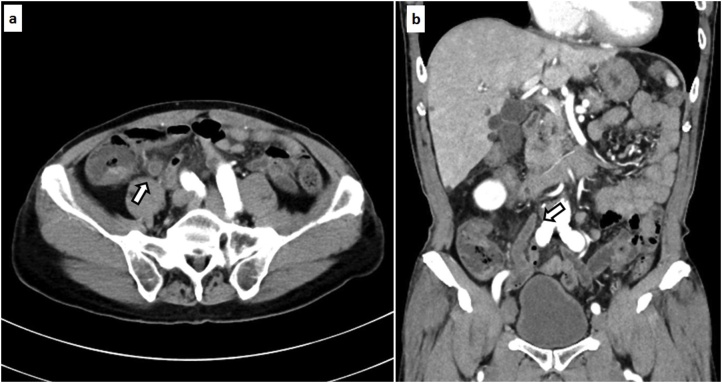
Contrast-enhanced CT demonstrated an enlarged appendix (10 mm in diameter) without fecalith, ascites, intraperitoneal free air, and abscess (appendix: thick white arrow). a) Axicial view, b) Coronal view.
